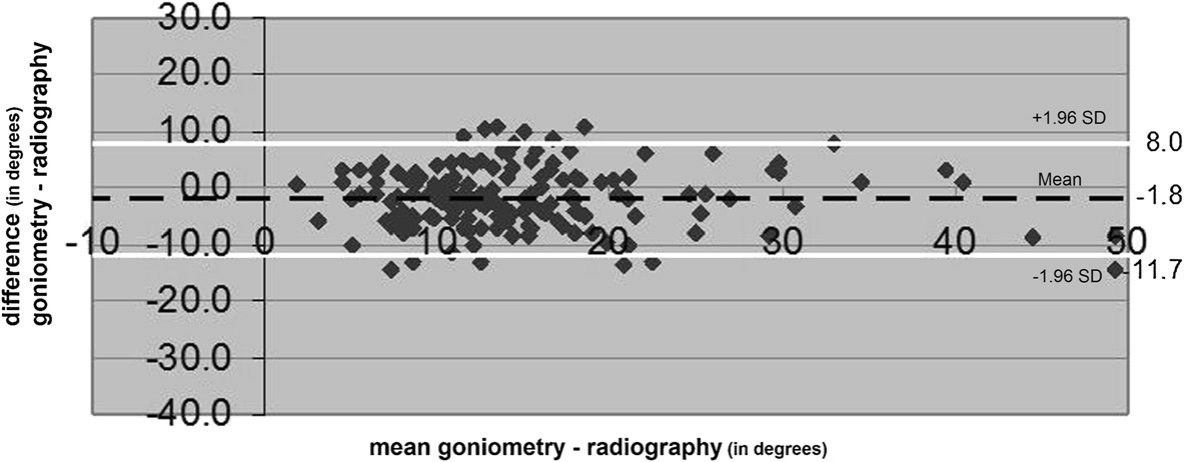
The range of the difference between the results of clinical goniometry of HVA and radiographic measurement of HVA. (Degrees). The mean difference (black discontinuous line) and the prediction interval (white lines) are presented.
